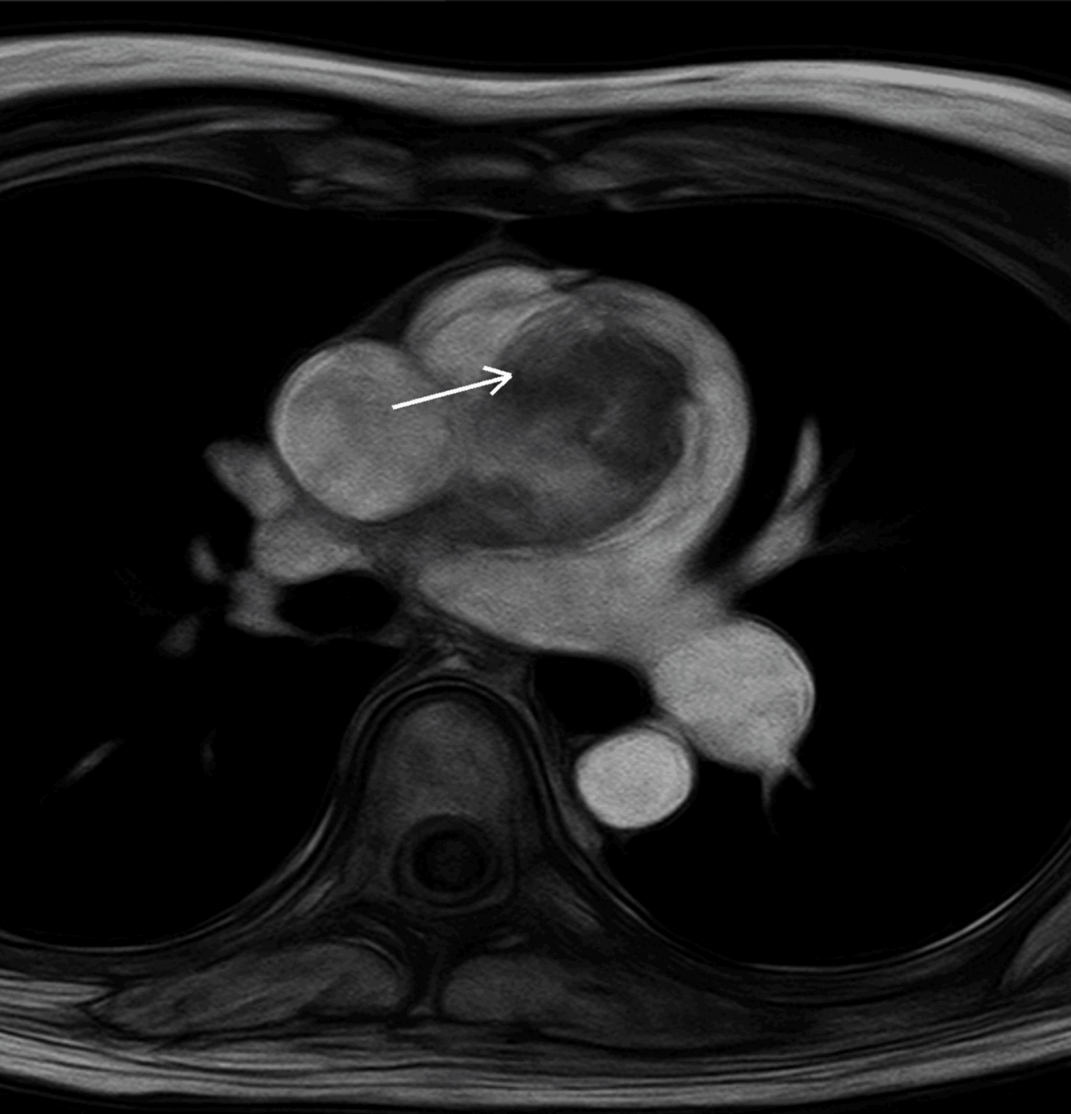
Magnetic resonance imaging of the pulmonary artery mass Axial magnetic resonance imaging demonstrates a large intraluminal soft-tissue mass within the main pulmonary artery with a broad-based attachment to the arterial wall.
